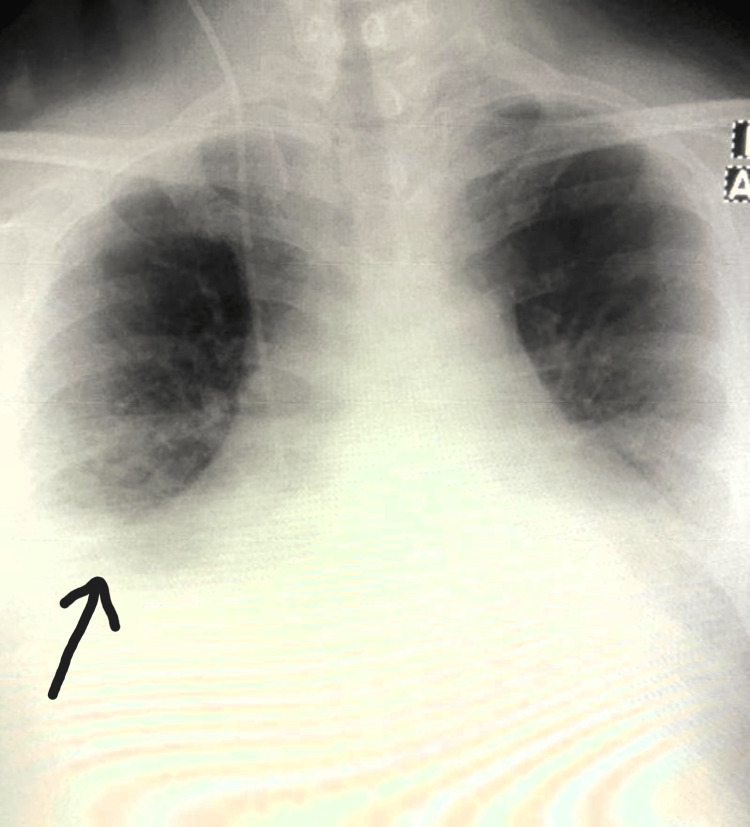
Chest x-ray showing right-sided pleural effusion and a bat wing appearance (a feature of pulmonary edema)
